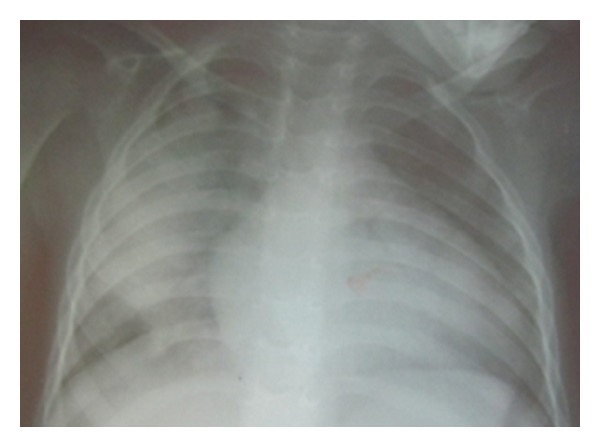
The thorax X-ray shows a cardiomegaly.
